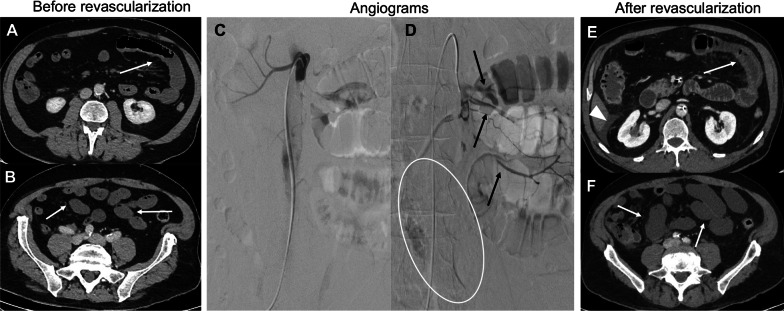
A 61-year-old male patient presenting with early acute mesenteric ischemia. The contrast-enhanced CT (portal venous phase) showed a decreased enhancement of the proximal jejunum (a) and ileum (b) wall. Digital subtraction angiography showed complete occlusion of the superior mesenteric artery (SMA) (c). Partial revascularization of the proximal jejunum arteries was achieved after 12 h of in situ thrombolysis with no opacification of the distal SMA (white oval) (d). Unfortunately, the patient experienced further clinical deterioration. Contrast-enhanced CT showed thickening of the jejunum wall with fat stranding (arrow) consistent with reperfusion injury following the recanalization of the jejunal arteries (e). Peri-hepatic fluid (arrowhead) was also noted. Additionally, CT showed a dilated jejunum with a persistent absence of wall enhancement, suggesting transmural necrosis of non-revascularized segments (f). The patient underwent a laparotomy that confirmed the absence of necrosis of the jejunum. He underwent subsequent resection of 225 cm of the small bowel and suffered from short bowel syndrome
